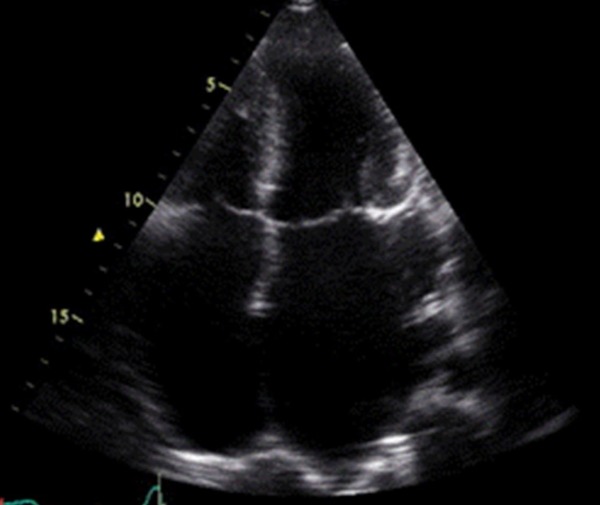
Echocardiogram in follow up demonstrating bi atrial enlargement
